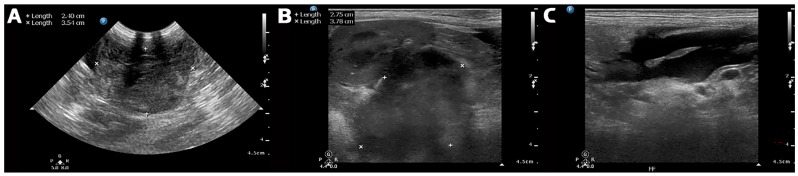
Focused abdominal ultrasonographic images of the abdominal lesion and hemoperitoneum. (A,B) Right-sided abdominal ultrasonographic views showing a hypoechoic mass located deep to the right kidney and anatomically associated with the right retroperitoneal region. (C) Focused abdominal ultrasonographic view showing free abdominal fluid consistent with hemoperitoneum.
